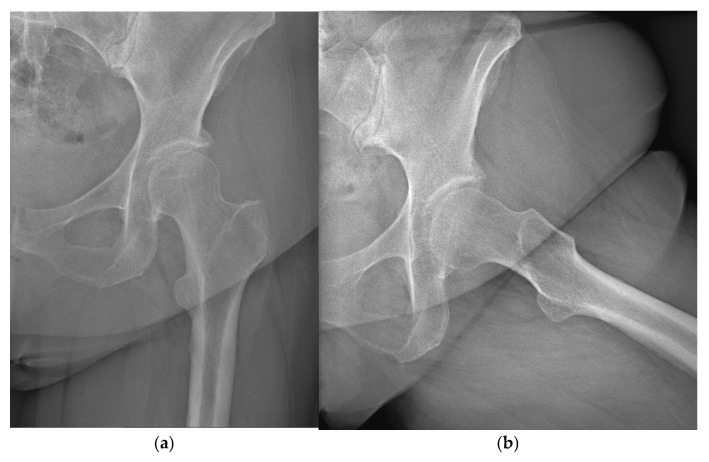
(a,b): Standard projections—preoperative anteroposterior and axial radiograph of a 58-year-old patient with secondary postdysplastic coxarthrosis of the left hip joint (Crowe type I). The narrow femoral canal (Dorr type A) is presented.
